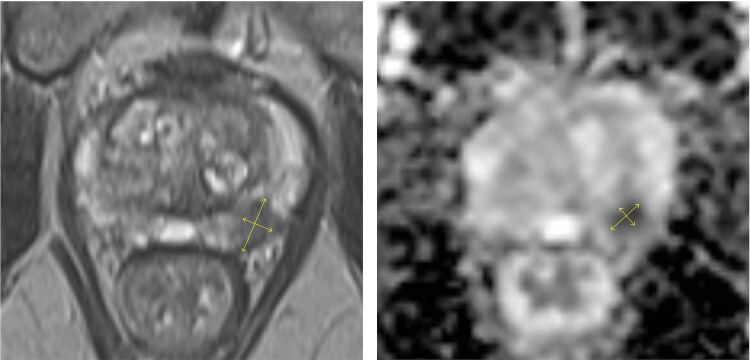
Multiparametric MRI of the prostate showing an 11 × 6 mm PI-RADS 4 lesion in the mid-third of the left dorsolateral peripheral zone with marked hypointensity on T2-weighted and diffusion-weighted images.
